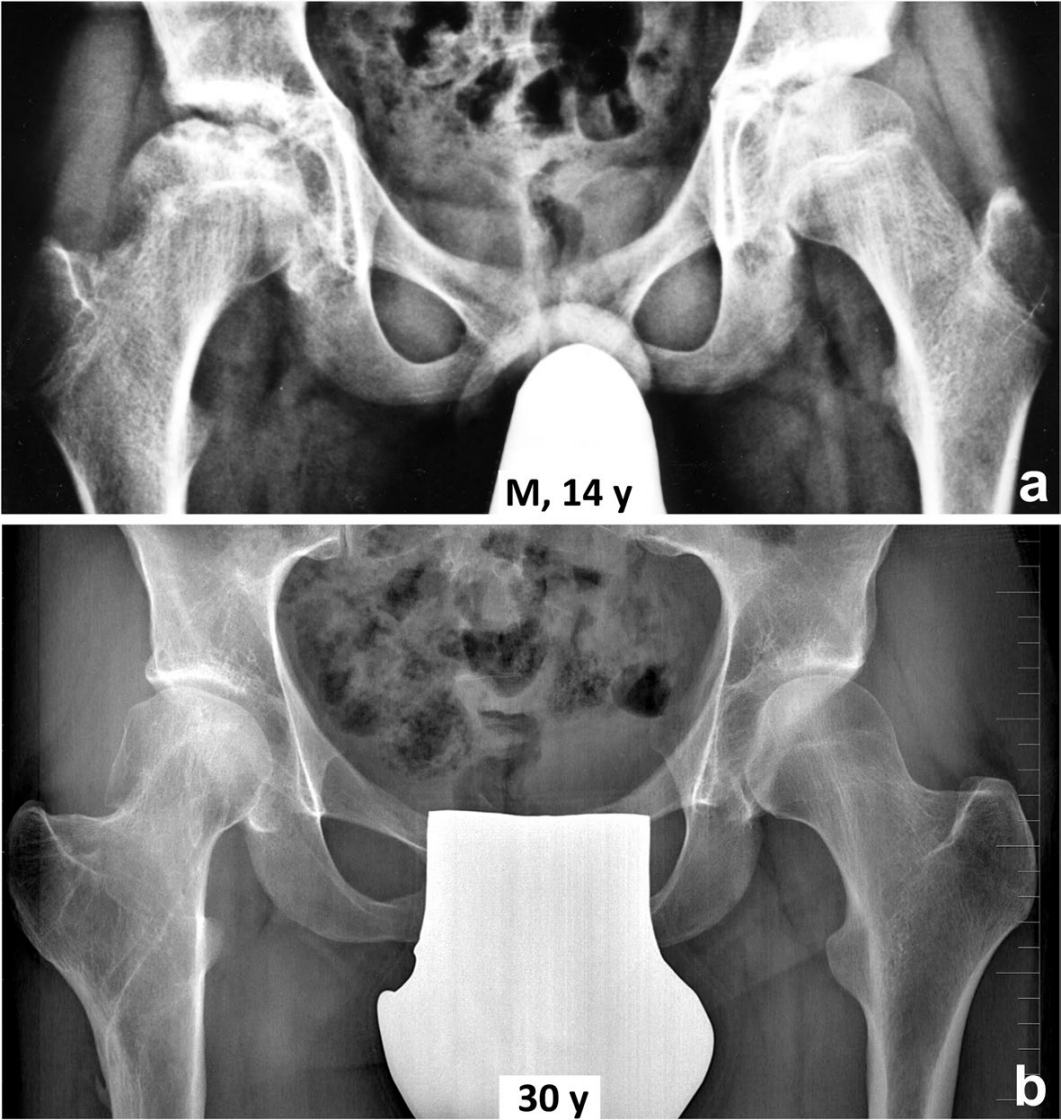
Patient N1 – the final result. a pelvic radiograph before surgery, b pelvic radiograph 16 years after surgery
